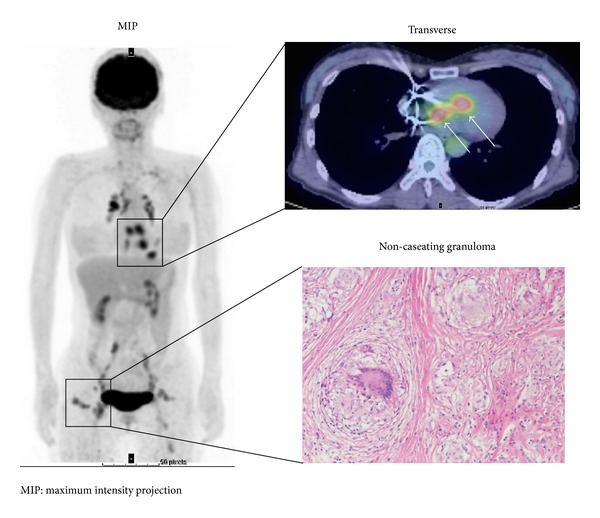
^18^F-FDG PET study in a subject with cardiac and extra-cardiac sarcoidosis. ^18^F-FDG uptake is focally increased in the basal septal wall, consistent with active CS, and is suppressed in other left ventricular walls (white arrows). Whole-body study shows multiple extra-cardiac foci of ^18^F-FDG uptake in chest, lymph nodes, and subcutaneous tissue consistent with active extensive systemic sarcoidosis. ^18^F-FDG PET: ^18^F-fluorodeoxyglucose positron emission tomography, MIP: maximum intensity projection.
